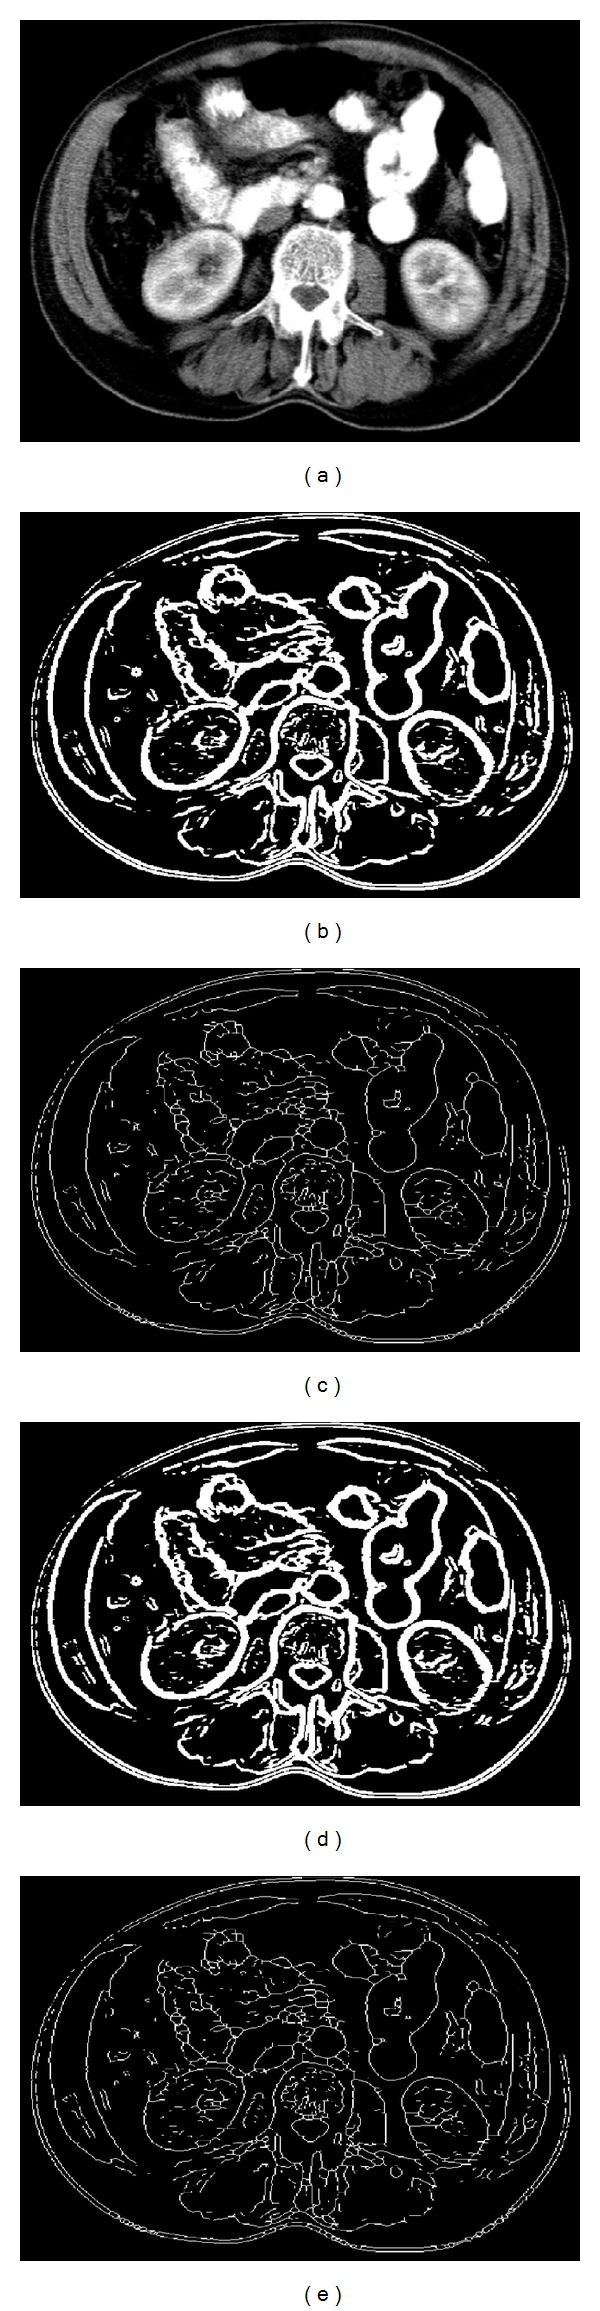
Edges of an abdominal CT image (*σ* = 0.18 and *ω* = 0.0005); (a) original, (b) *k*-means, (c) skeleton of (b), (d) FCM, (e) skeleton of (d).
